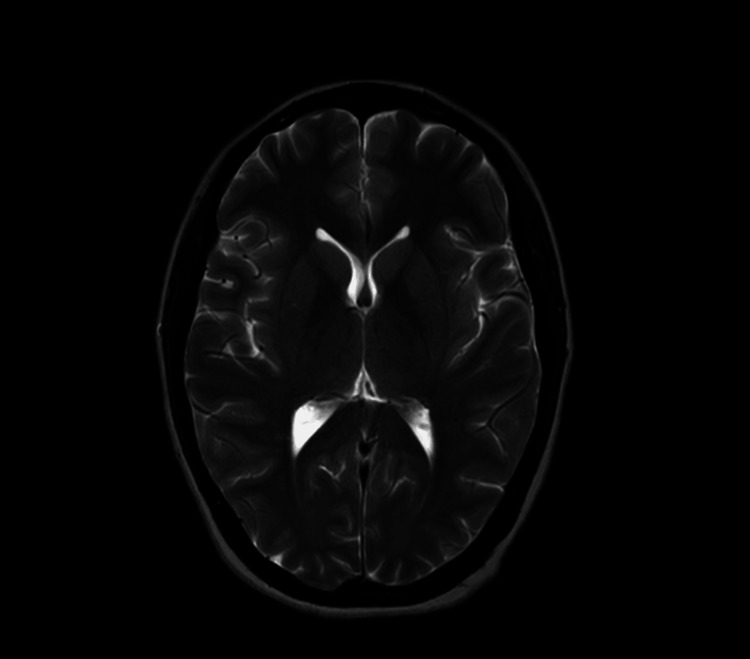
Magnetic resonance imaging (MRI) brain of the patient showing no brain parenchymal abnormalities, supporting the diagnosis of functional neurological symptom disorder (FND) with no underlying organic cause
